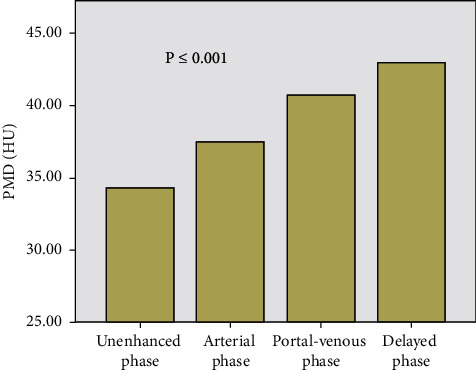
In different phases of enhanced CT, the PMD value gradually increased, and the difference was statistically significant.
